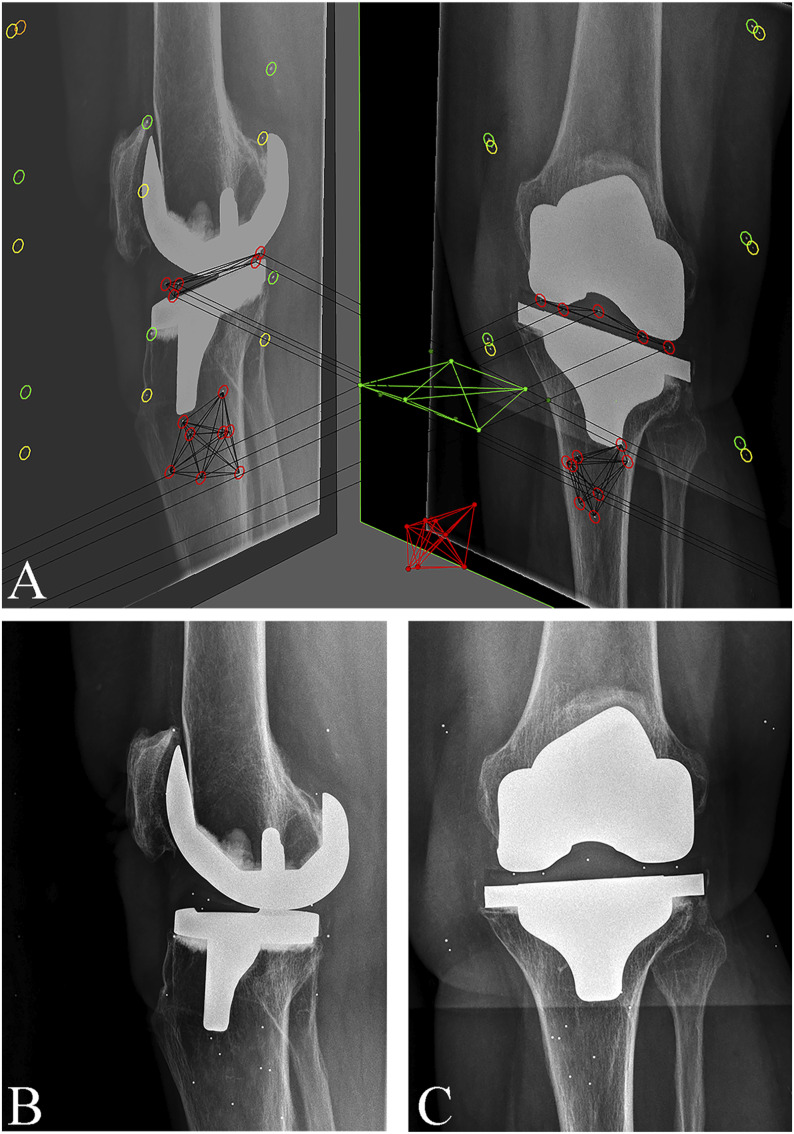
Figs. 4-A, 4-B, and 4-C RSA images of a cemented TKA implant. Fig. 4-A Biplanar (lateral and anteroposterior) views with markers inserted in the polyethylene insert and tibial bone. Fig. 4-B Lateral radiograph of the same implant, which was classified as continuously migrating. Fig. 4-C Anteroposterior radiograph of the same implant.
